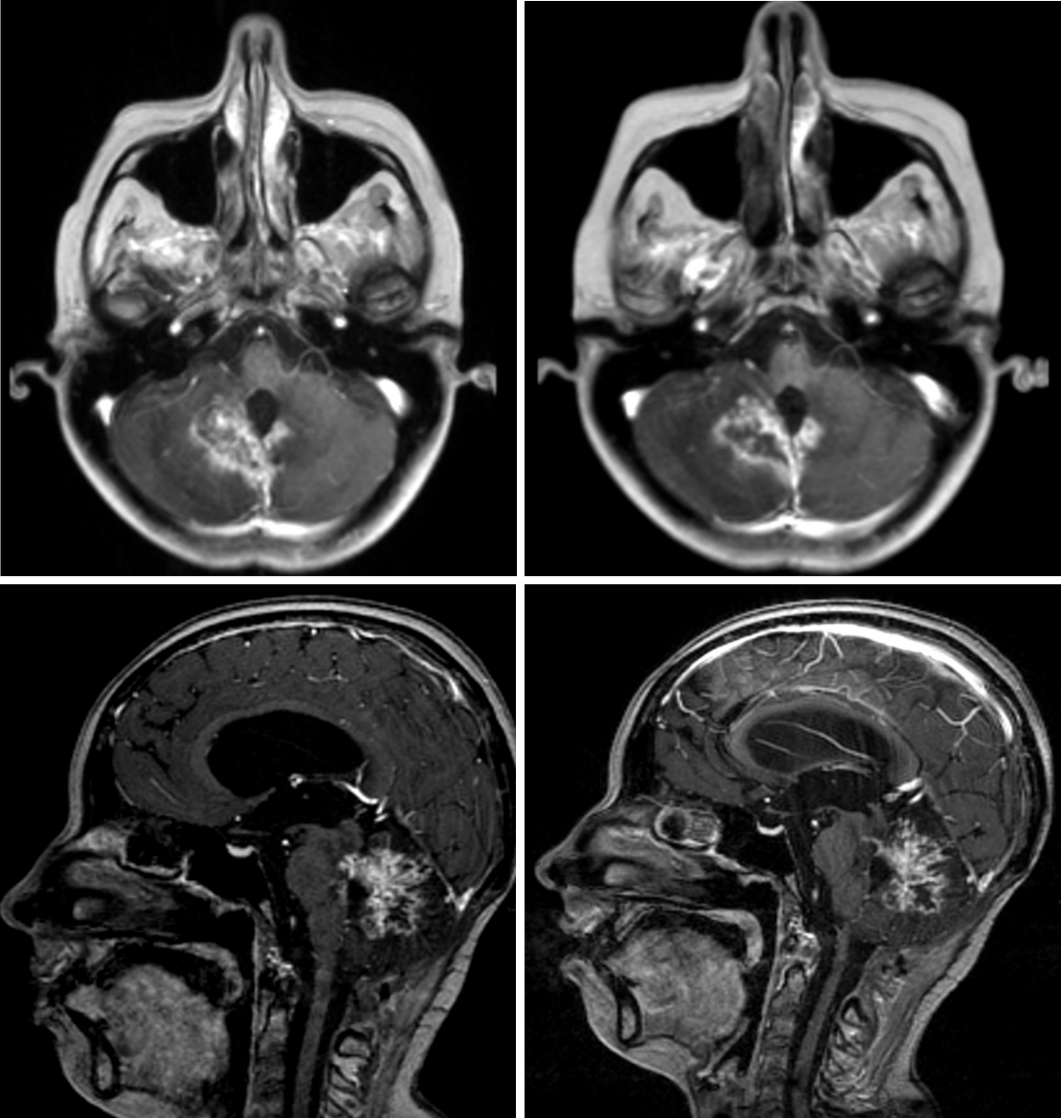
Stable disease in magnetic resonance imaging (MRI) during octreotide treatment Left (Top and Bottom): Axial and sagittal view in February 2014 Right (Top and Bottom): Axial and sagittal view in May 2015
